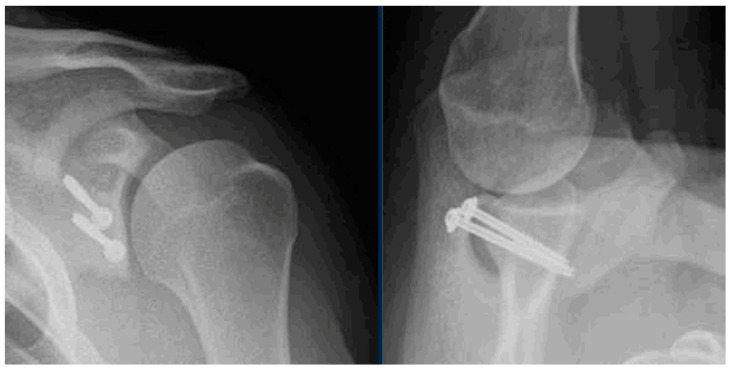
Grashey and axillary radiographs are obtained post-operatively. The XRs shown demonstrate appropriate position of the graft, flush with the native glenoid rim.
